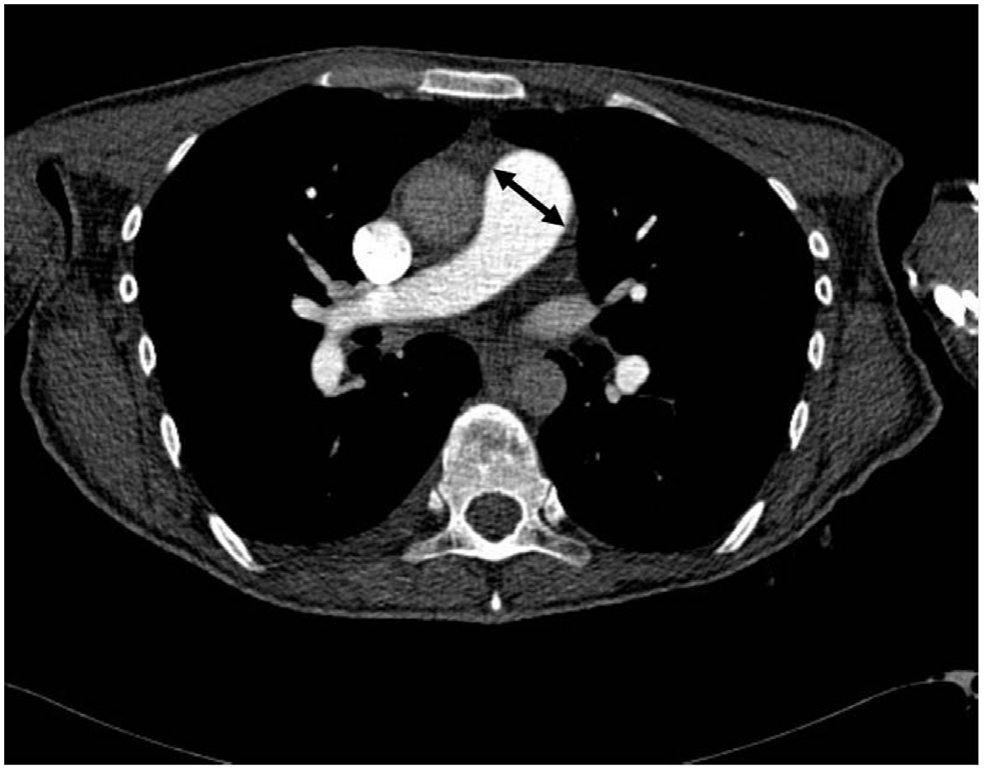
The pulmonary artery diameter was measured at the trunk of the pulmonary artery, on axial CT angiography slices using electronic callipers.
